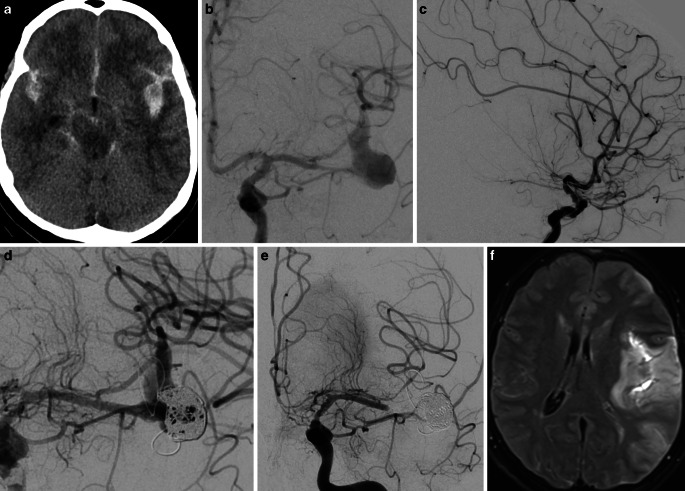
A case of 57-year-old female patient who presented with acute SAH (a), Hunt and Hess II due to fusiform, most likely dissecting aneurysm of the inferior trunk of the left MCA (b). Temporary occlusion of the parent vessel proximal to the aneurysm demonstrated insufficient leptomeningeal collaterals (c). Reconstructive treatment with partial coiling and telescopic deployment of three FDS from proximal to distal was performed (d). Stent thrombosis and refractory vasospasm (e) resulted in a large MCA infarction (f) and eventually death. a axial non-contrast computer tomography (NCCT), b frontal DSA after left internal carotid artery (ICA) injection, c lateral DSA after left ICA injection with inflated balloon in the inferior trunk of the MCA, d final run after partial coiling and flow diversion, e frontal left ICA injection 9 days after treatment, f axial FLAIR (fluid attenuated inversion recovery)
